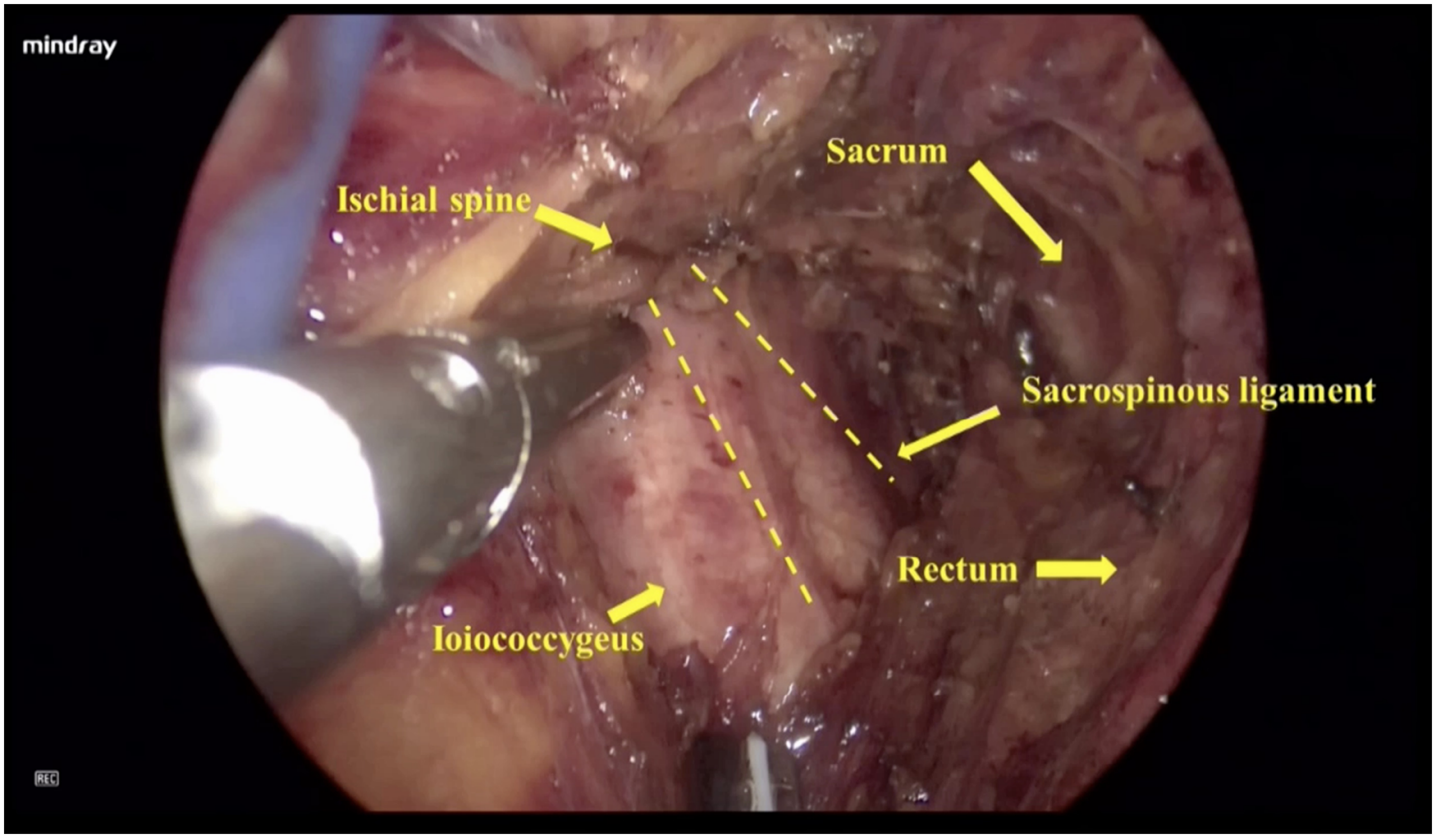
Sacrospinous ligament exposure. An ultrasonic scalpel was used to laparoscopically separate the loose tissues next to the rectum until the sacrospinous ligament, the adjacent piriformis, and iliococcygeal muscles were fully exposed.
